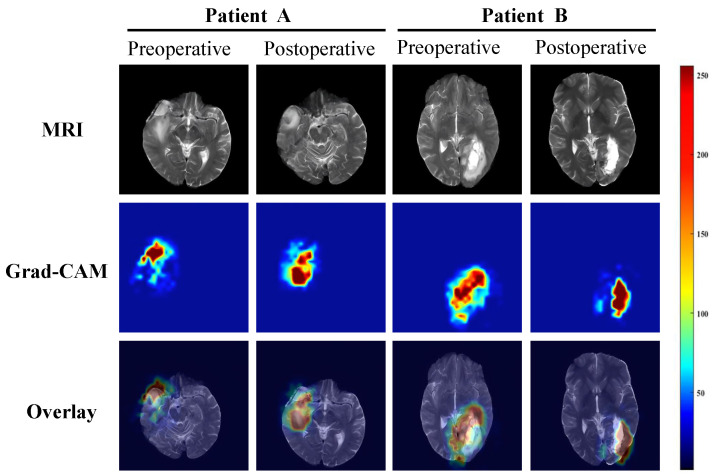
Example of Grad-CAM visualization of the Mamba-Diff Net model. It shows preoperative and postoperative MRI images of a typical case and the corresponding model attention areas.
